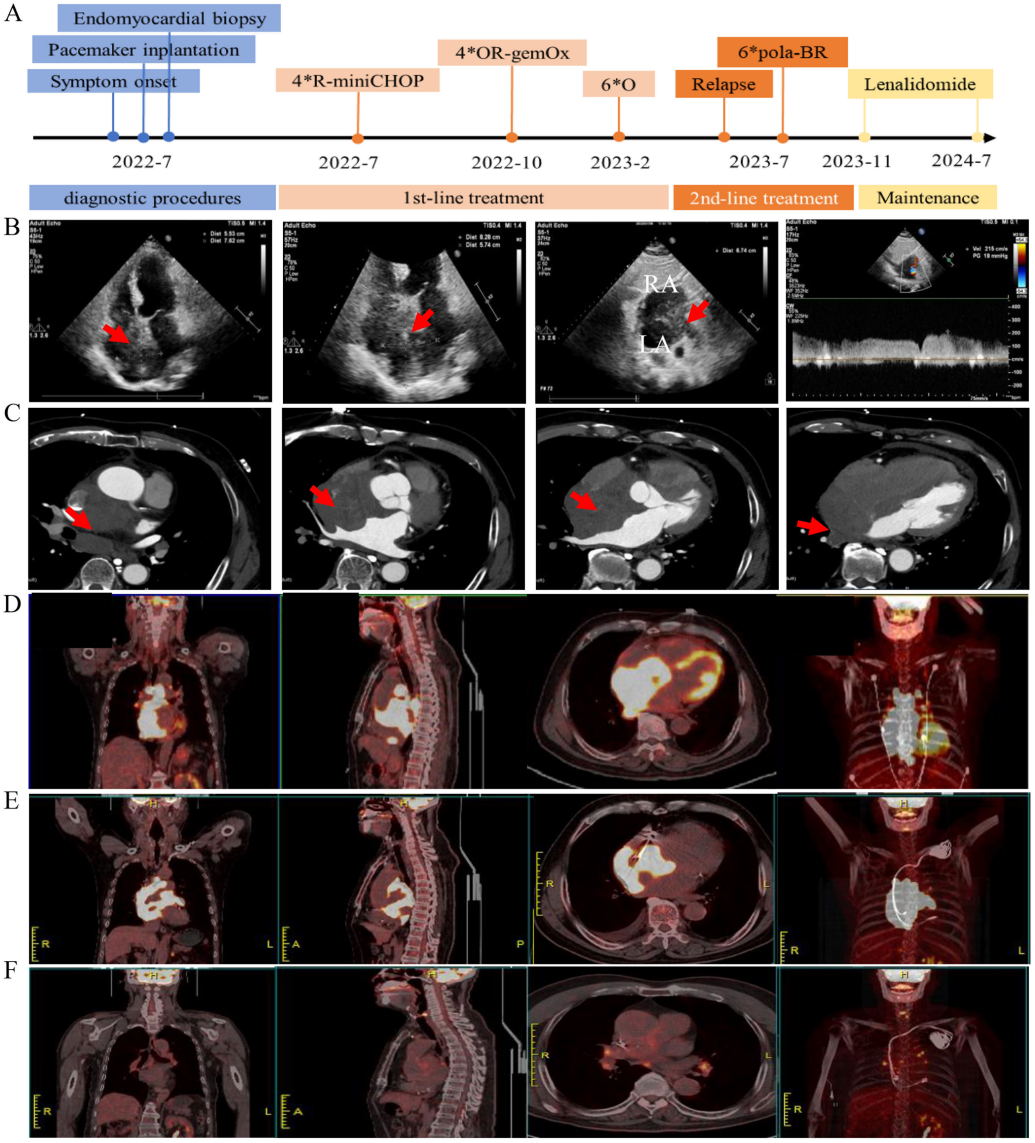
Radiological imaging before and after the treatment. (A) Timeline of clinical events, and interventions. (B) Transthoracic echocardiogram showing a large mass (red arrow) infiltrating the interatrial septum. Panel 1–3 show the apical 4-chamber and subxiphoid two-chamber views of the interatrial septal mass, panel 4 shows pulse-wave doppler imaging of the mass at the junction of the superior vena cava and the right atrium with increased blood flow velocity. (C) Contrast-enhanced CT scan shows a large mass (red arrow) in the middle mediastinum invading the left and right atria. (D) PET/CT shows increased 18-FDG-uptake heart masses invading surrounding structures at diagnosis. (E) PET/CT shows increased 18-FDG-uptake heart masses at relapse. (F) PET/CT shows no evidence of lymphoma after treatment for disease relapse.
